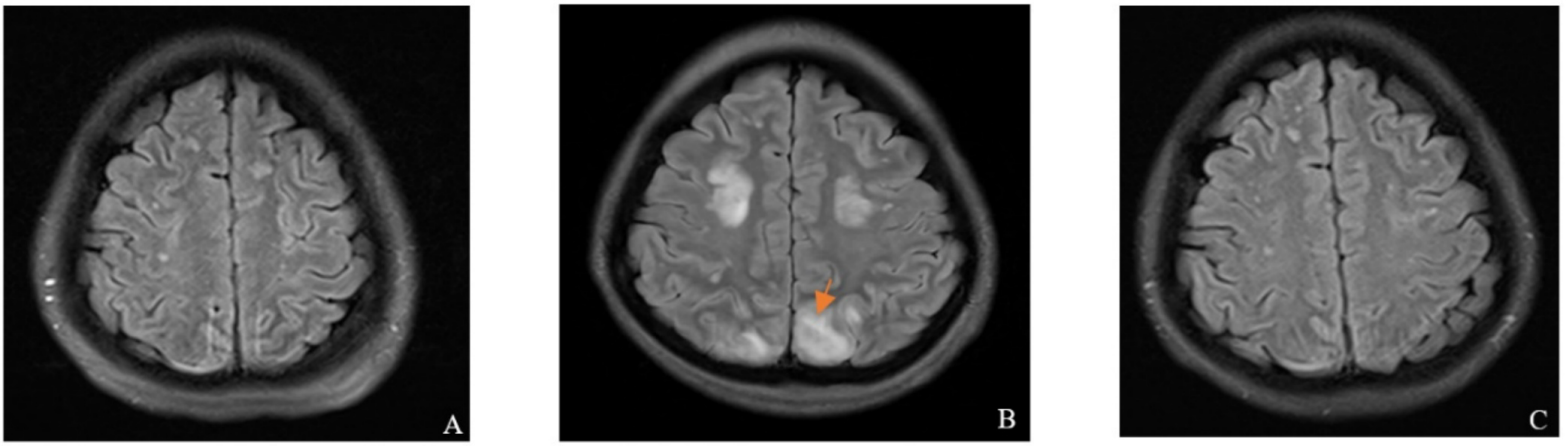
Brain MRI findings of the patient. (A) On admission, the MRI showed multiple abnormal signals in the brain, and microvascular thrombosis could not be ruled out. (B) Ten days after admission, the MRI revealed multiple abnormal signals in the brain (Where the orange scissors point), with a high likelihood of PRES. (C) After four weeks of eculizumab treatment, the MRI indicated multiple abnormal signals in the brain, which were strongly suggestive of PRES; however, the lesions showed improvement compared with the previous MRI results. MRI, magnetic resonance imaging; PRES, posterior reversible encephalopathy syndrome.
